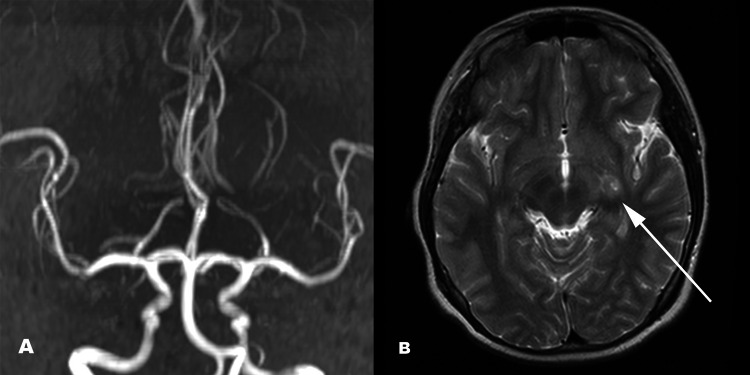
Follow-up MRI showing MR angiography (A) and axial-cut T2-weighted image (B). No stenosis, beaded appearance, or abnormal signal intensity from the vessel can be seen on MRA (A), particularly on the left side. Retrospectively, small cystic lesions were noted in the left basal ganglia (B, white arrow). There was also persistent mild asymmetry of the lateral ventricle, along with a slightly larger left lateral ventricle (not shown). MRA: magnetic resonance angiography
